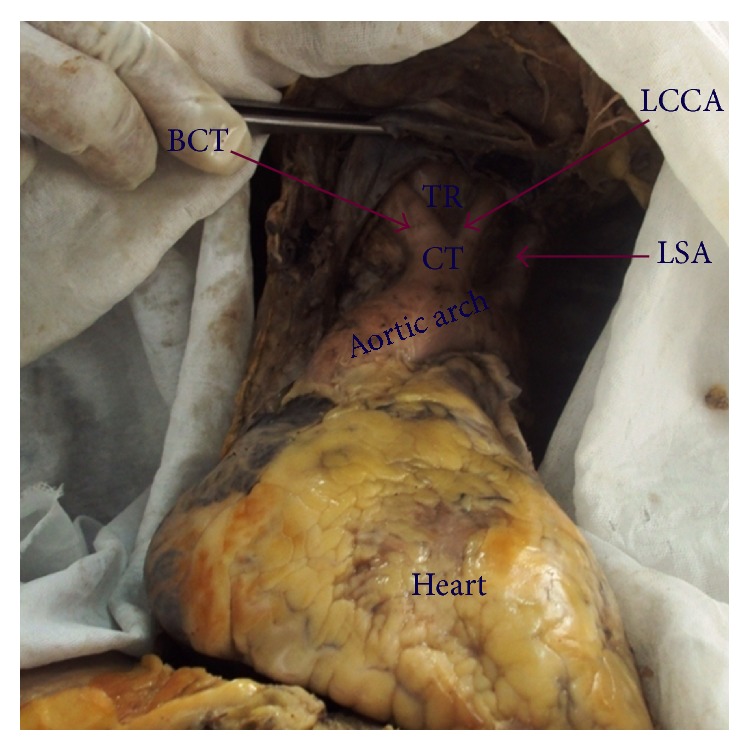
Aortic arch showing two branches (common trunk for brachiocephalic trunk and left common carotid artery). CT: common trunk, BCT: brachiocephalic trunk, LCCA: left common carotid artery, LSA: left subclavian artery, and TR: trachea.
